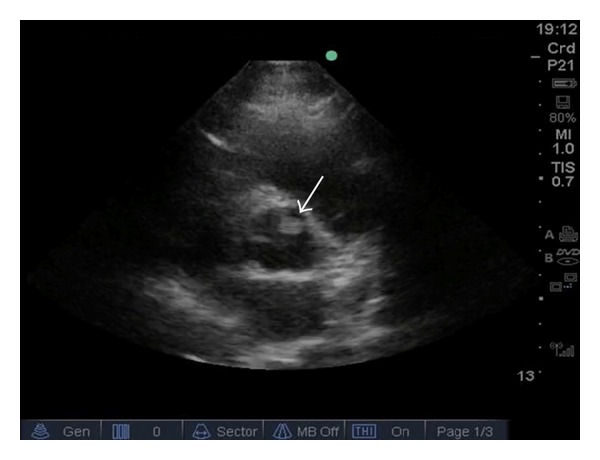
Ultrasound parasternal short axis view showing the aortic valve with the circular well circumscribed hyperechoic area, consistent with a vegetation or valvular mass.
